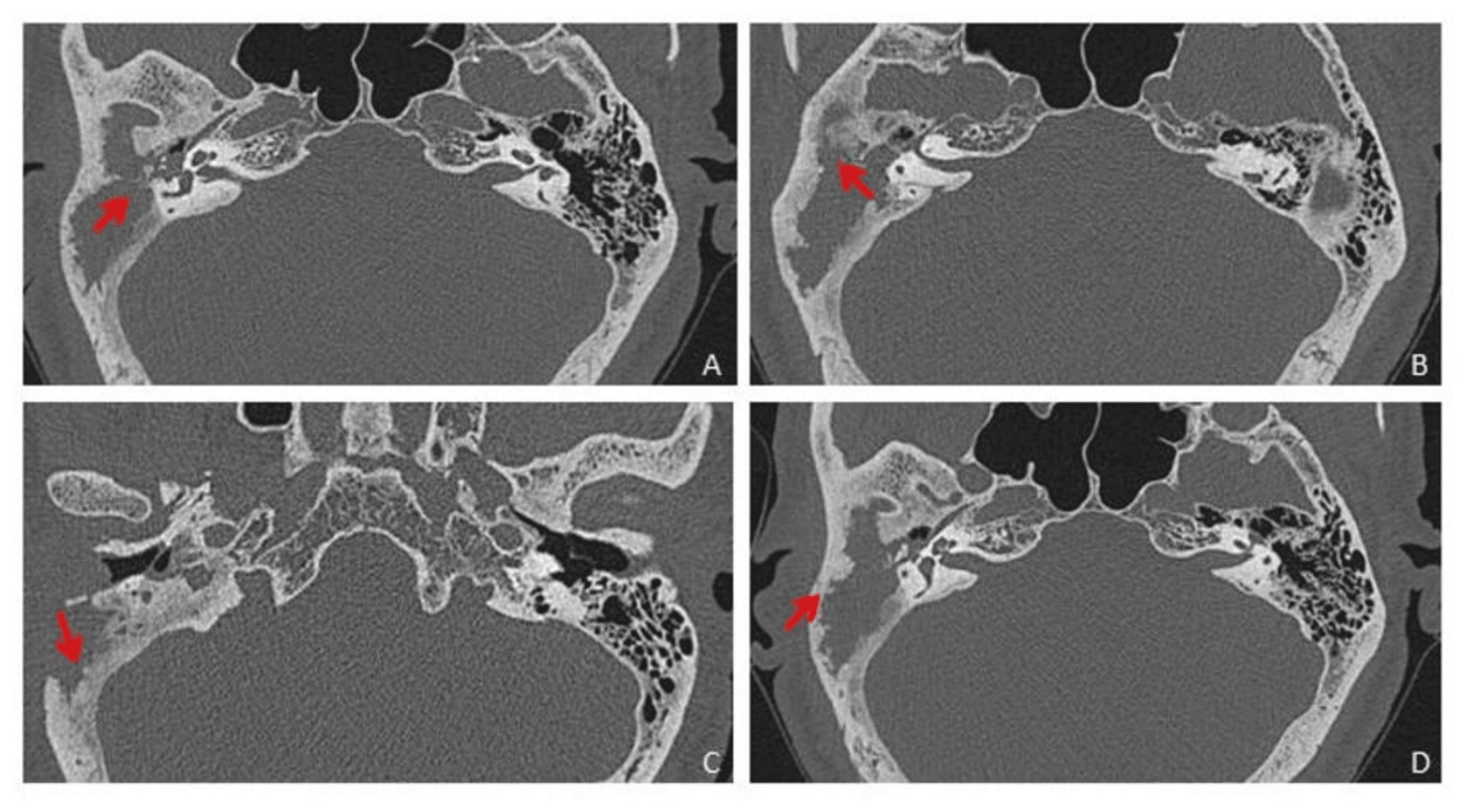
HRCT temporal axial images showing soft tissue density lesion in the right middle ear cavity and mastoid air cells with the destruction of the mastoid septae, outer mastoid cortex and adjoining bony external auditory canal. HRCT: High-resolution computed tomography
